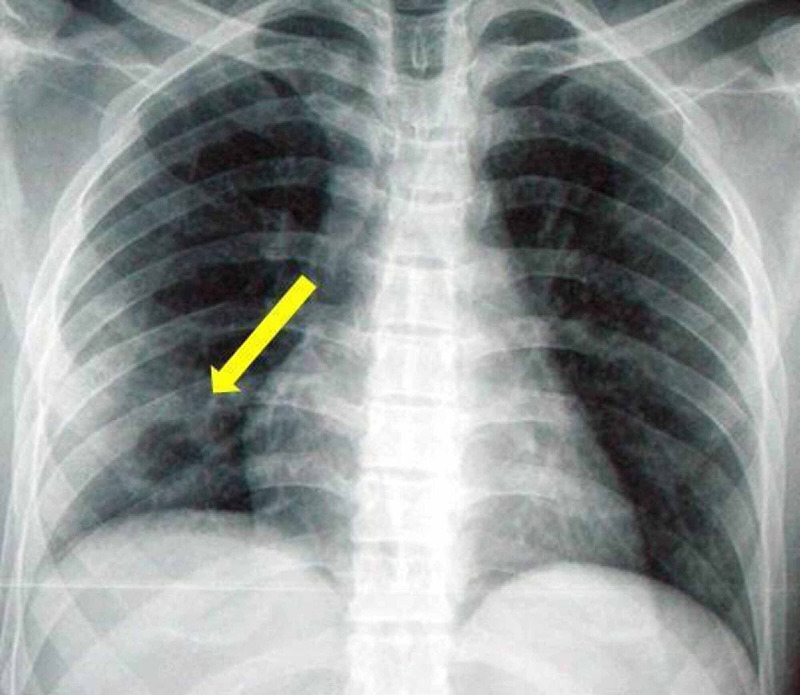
Chest X-ray showed a round and excavated lesion (arrow).
